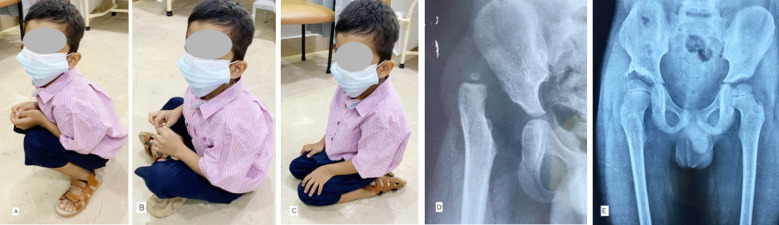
A 30-month-old boy, 30 months after open reduction and Pemberton’s Pelvic osteotomy of right side DDH. Siting at ease in position of Squat Type-I (A), Palthi Type-I (B) & Tashahhud Type-I (C). BFHS Score Excellent. Pre-operative Xray show Tonnis IV dislocation (D), Post-operative Xray show Severin class1a (E). Clinico-radiological score Excellent.
